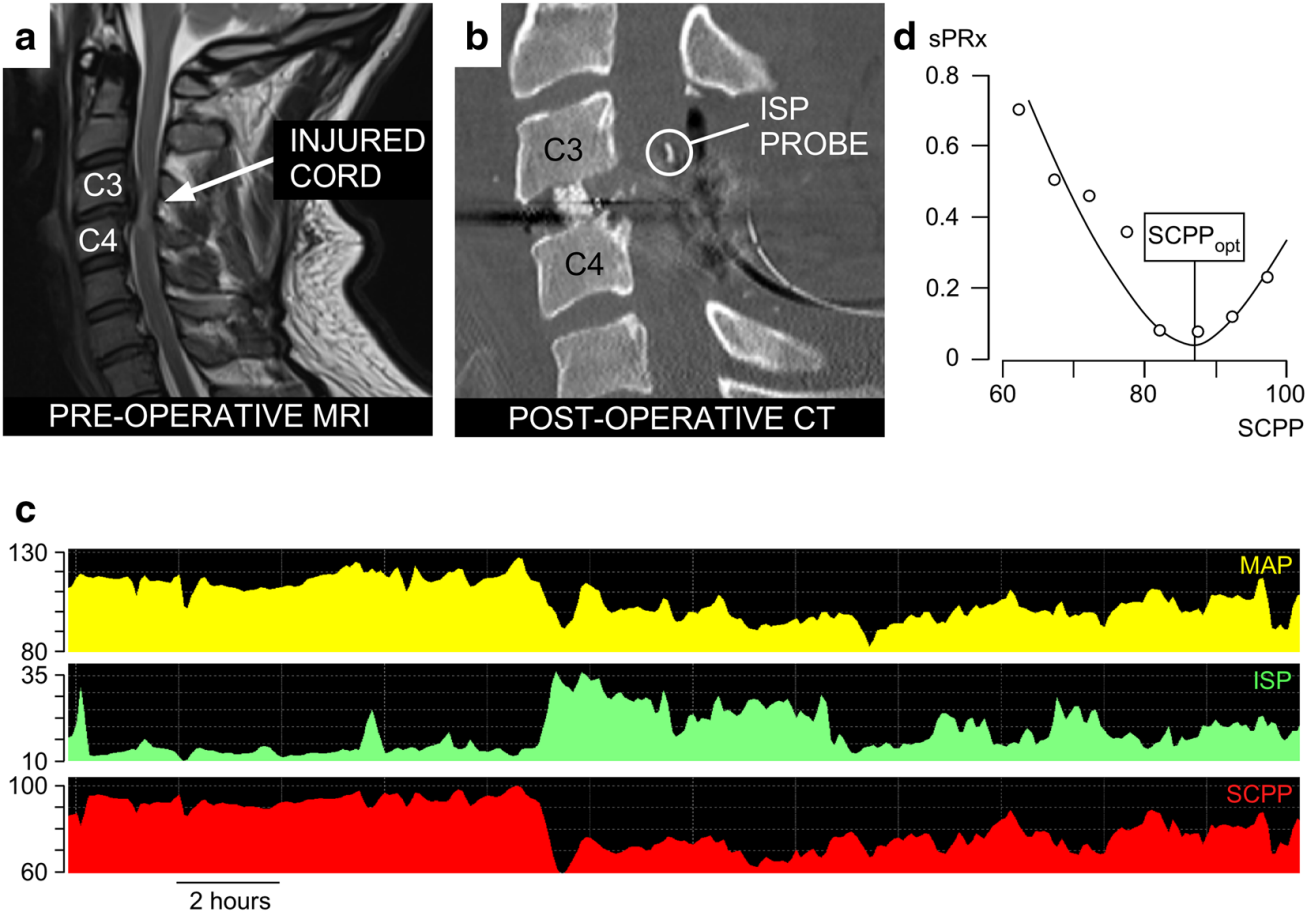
ISP monitoring technique. a Preoperative MRI of a 37-year-old male patient with TSCI AIS grade C at C3/4. b Postoperative CT of same patient showing C3/4 anterior cervical cage, posterior C3/4 laminectomies, and ISP probe (circled). c MAP, ISP SCPP signals. d sPRx versus SCPP plot. Minimum is SCPP_opt_
